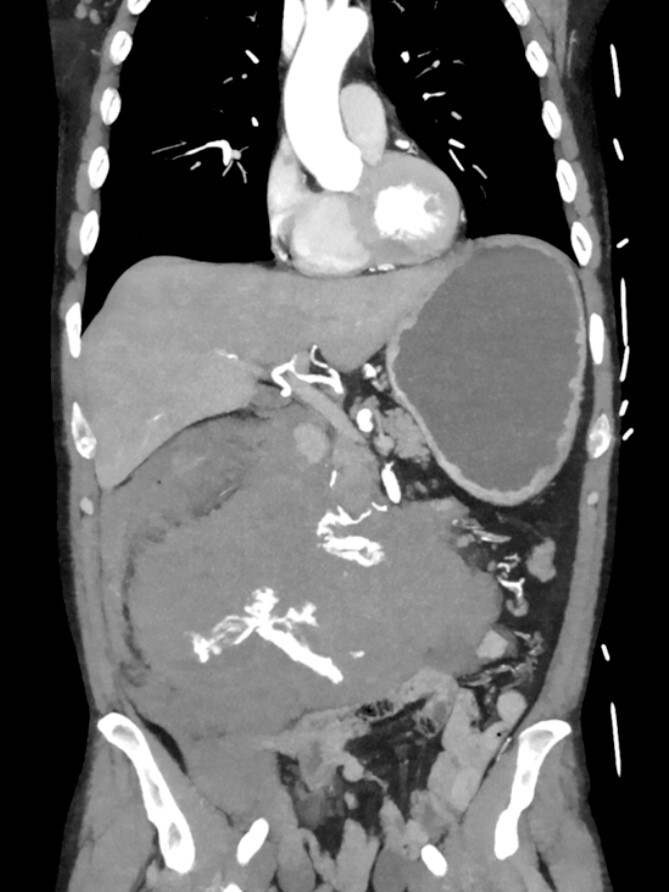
CTA showing an active bleeding likely from the pancreaticocuodenal artery with venous pooling and a large retroperitoneal hematoma.
